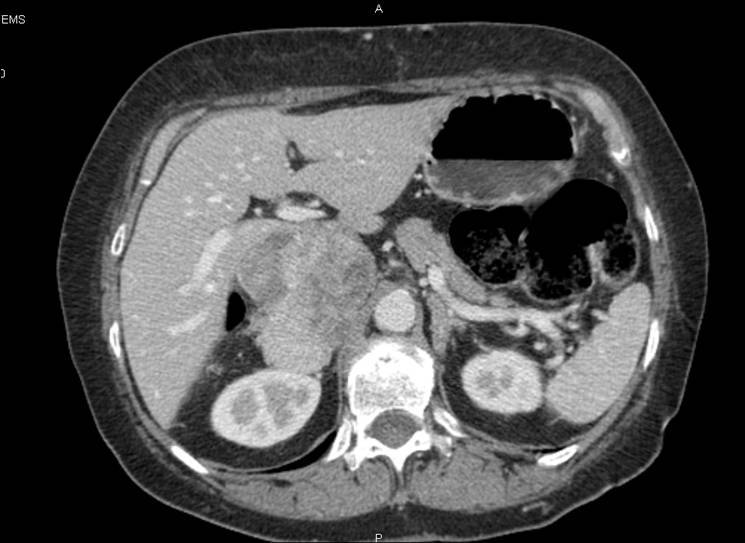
Pre-operative CT—patient 8.
